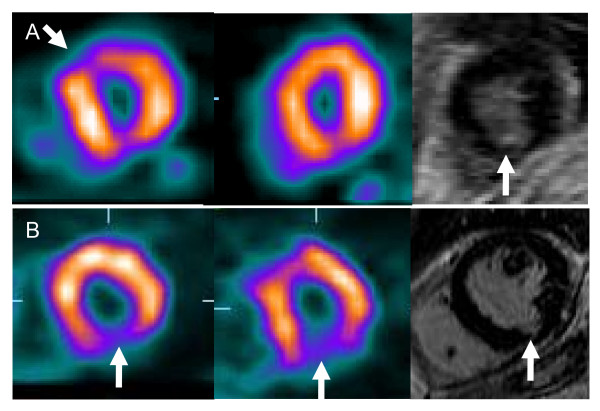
Two patients with myocardial infarction on CMR. A. A 74-year old woman with an inferior subendocardial infarction. The minor inferior perfusion defect was not read as a true defect, but the patient was included because of the anterior defect in supine imaging. The infarct did not cause decreased function on gated SPECT. B. A 69-year old man with an inferior subendocardial infarction detected on prone and supine SPECT as a perfusion defect.
